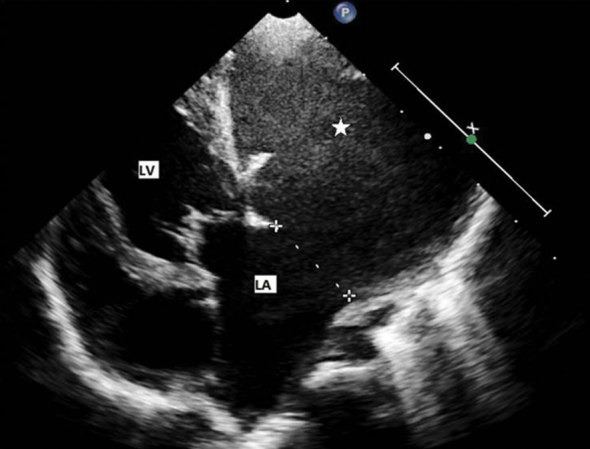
Transthoracic echocardiography (four-chamber view). Echocardiography shows a large left paracardiac lesion (star) widely communicating with the LA. LA, left atrium; LV, left ventricle.
